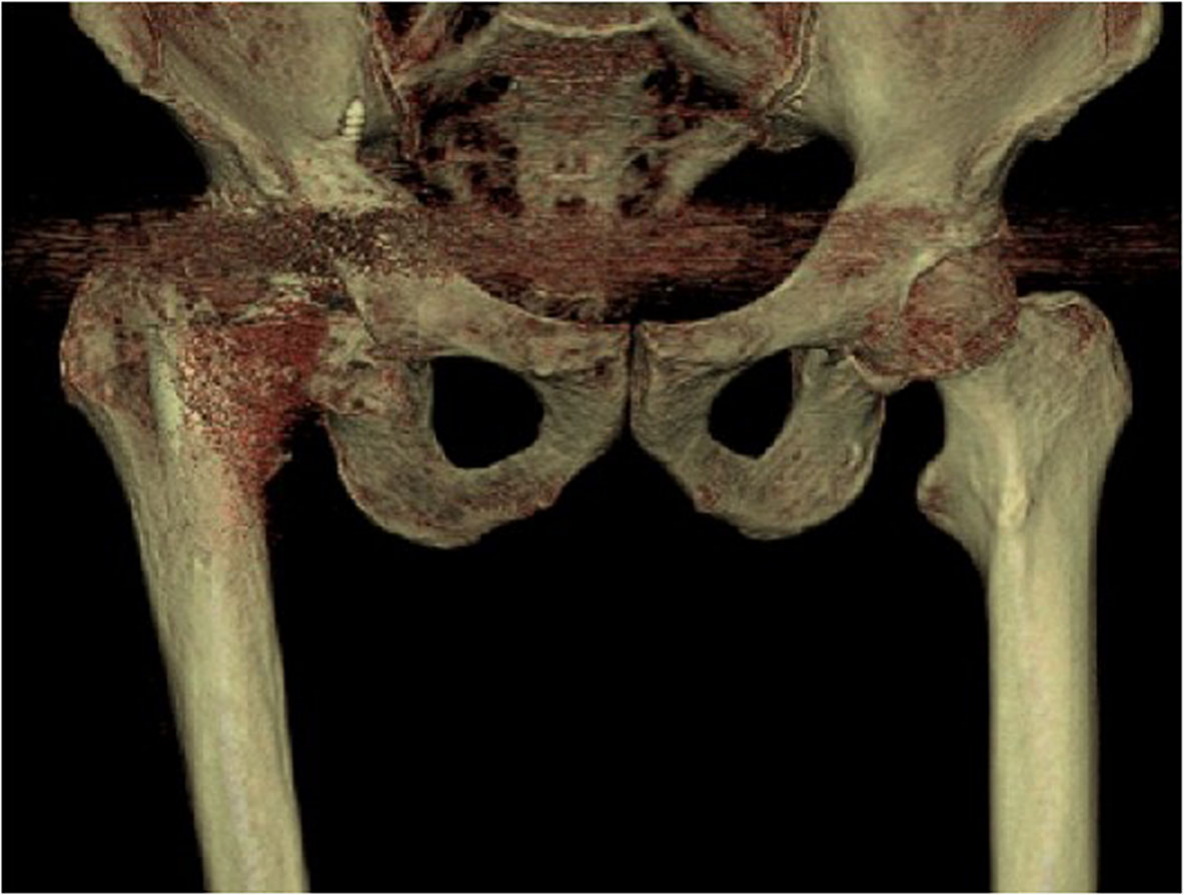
An AL patient’s 3D CT imaging
